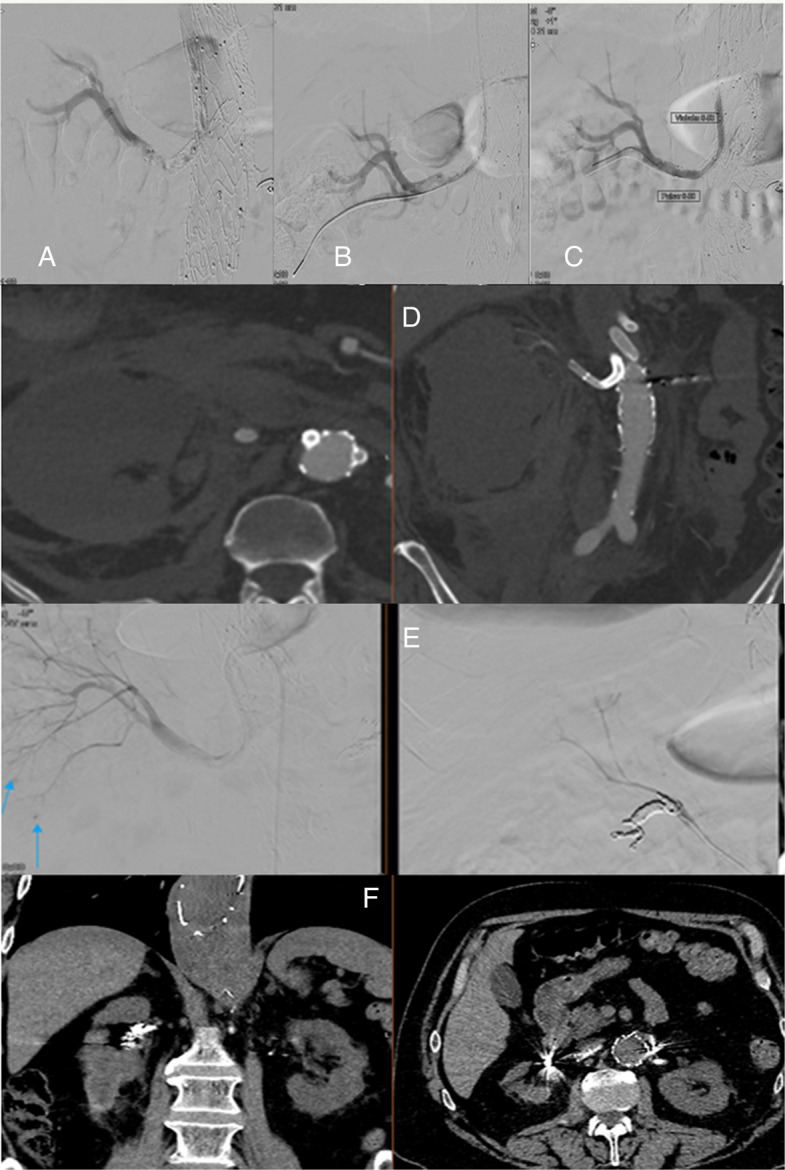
The same patient shown in Fig. 1 became hemodynamically unstable a few hours after the procedure. A Right renal DSA after recanalisation shows residual thrombus within the stent and no flow distal to the segmental arteries. B The tip of the catheter is in a distal-pole segmental branch. C After withdrawal of the catheter or a guidewire, a significant narrowing is seen at the proximal part of the renal artery stent graft with no evidence of blood flow distal to the segmental arteries. D CT shows renal and perirenal hematoma with no active contrast extravasation. There is no blood flow in the intra-renal arterial branches. E Angiography was performed, and embolisation of the lower pole of the right kidney was performed with 3 mm and 5 mm microcoils. The patient required temporary dialysis but eventually recovered. At present, the patient is off dialysis, with severely impaired renal function (eGFR 17 mL/min) and preserved diuresis. F A follow-up non-contrast CT scan demonstrated a reduction in renal parenchymal volume
